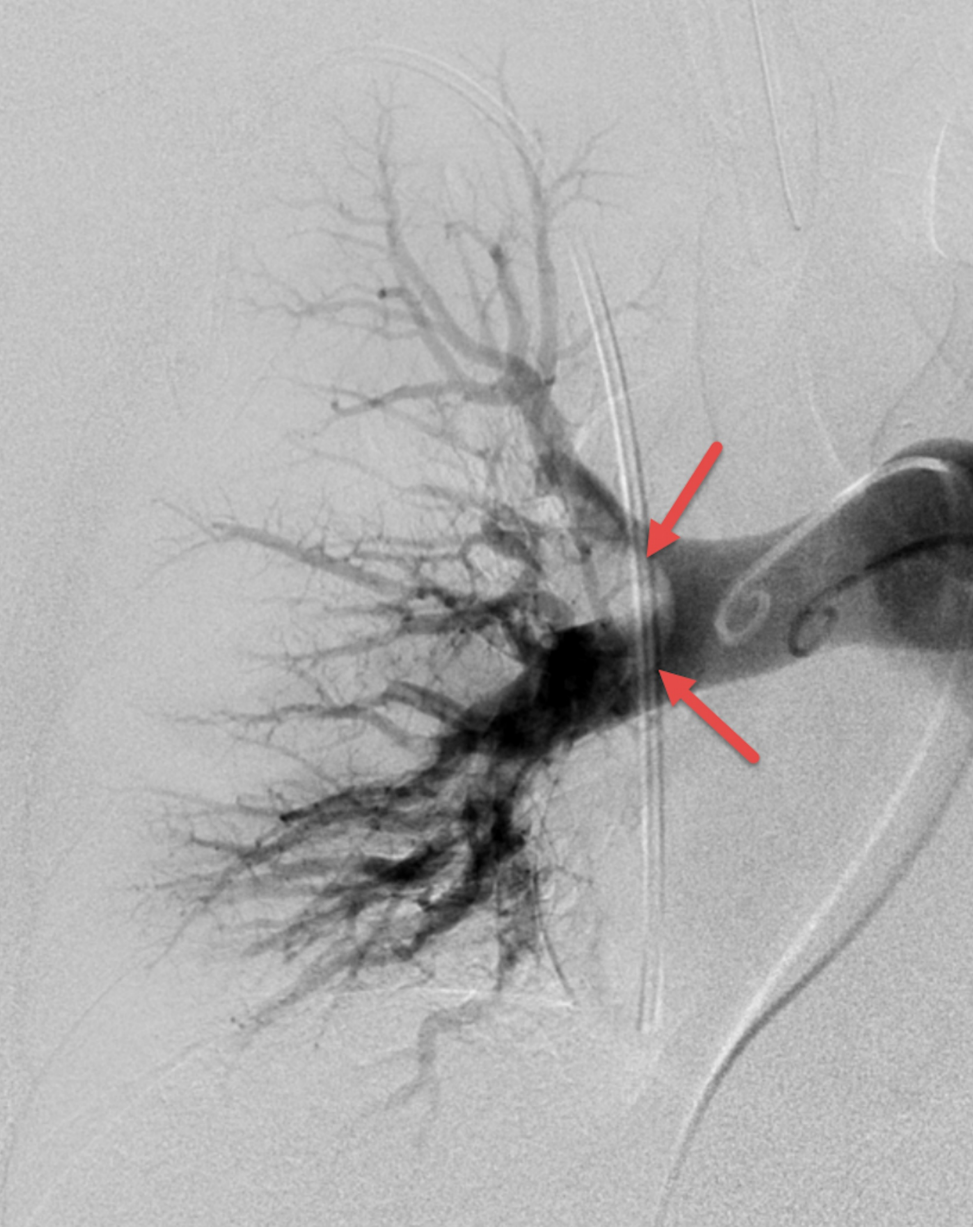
Right Lung Pre-Mechanical Embolectomy CT Angiography Showing the Meniscus of the Large Embolus in the Lower Lobe.
